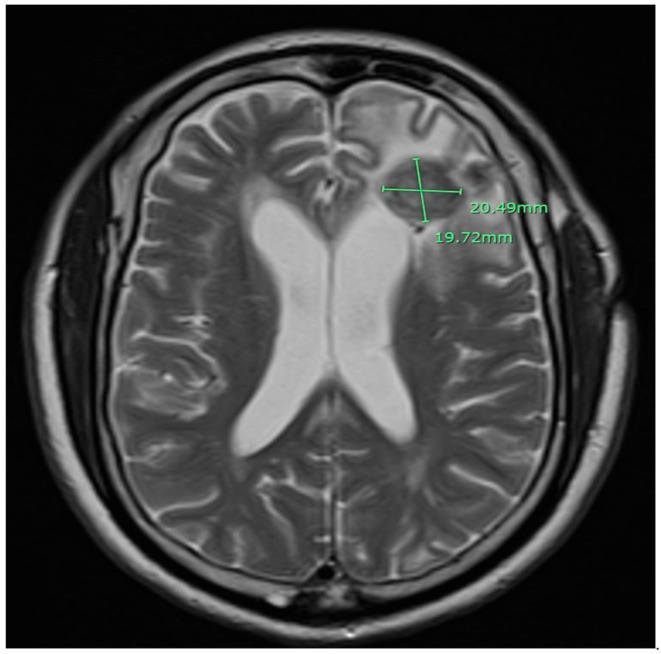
Control magnetic resonance imaging (MRI) of the brain with gadolinium showed 20 mm × 19 mm left frontal lobe mass is significantly decreased in size relative to the previous examination and does not demonstrate the significant mass effect.
